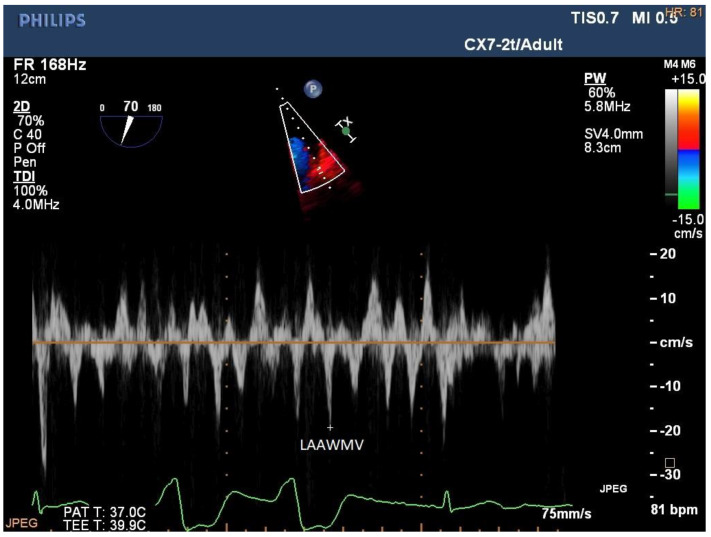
Left atrial appendage wall motion velocity (LAAWMV). LAAWMV 18 cm/s. Pulsed-wave tissue Doppler in two-chamber 60–90° projection in transoesophageal echocardiography during atrial fibrillation.
